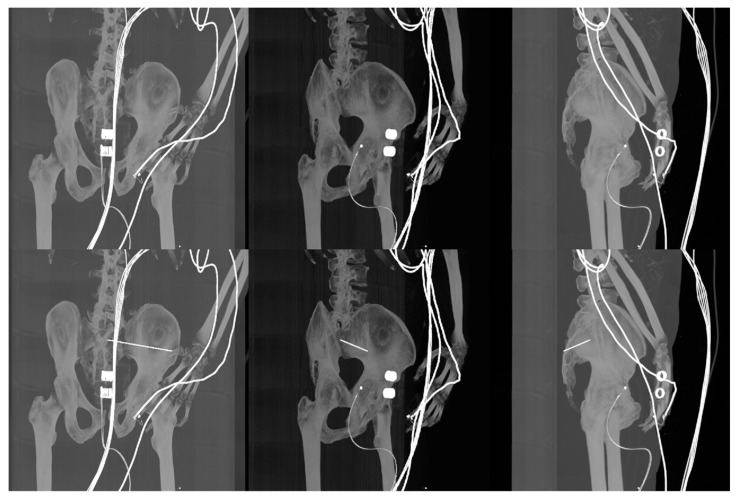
Comparison of three various CT slices (Top Row) of patient 2 with the same DDR projections with virtual iliosacral screw (Bottom Row).
